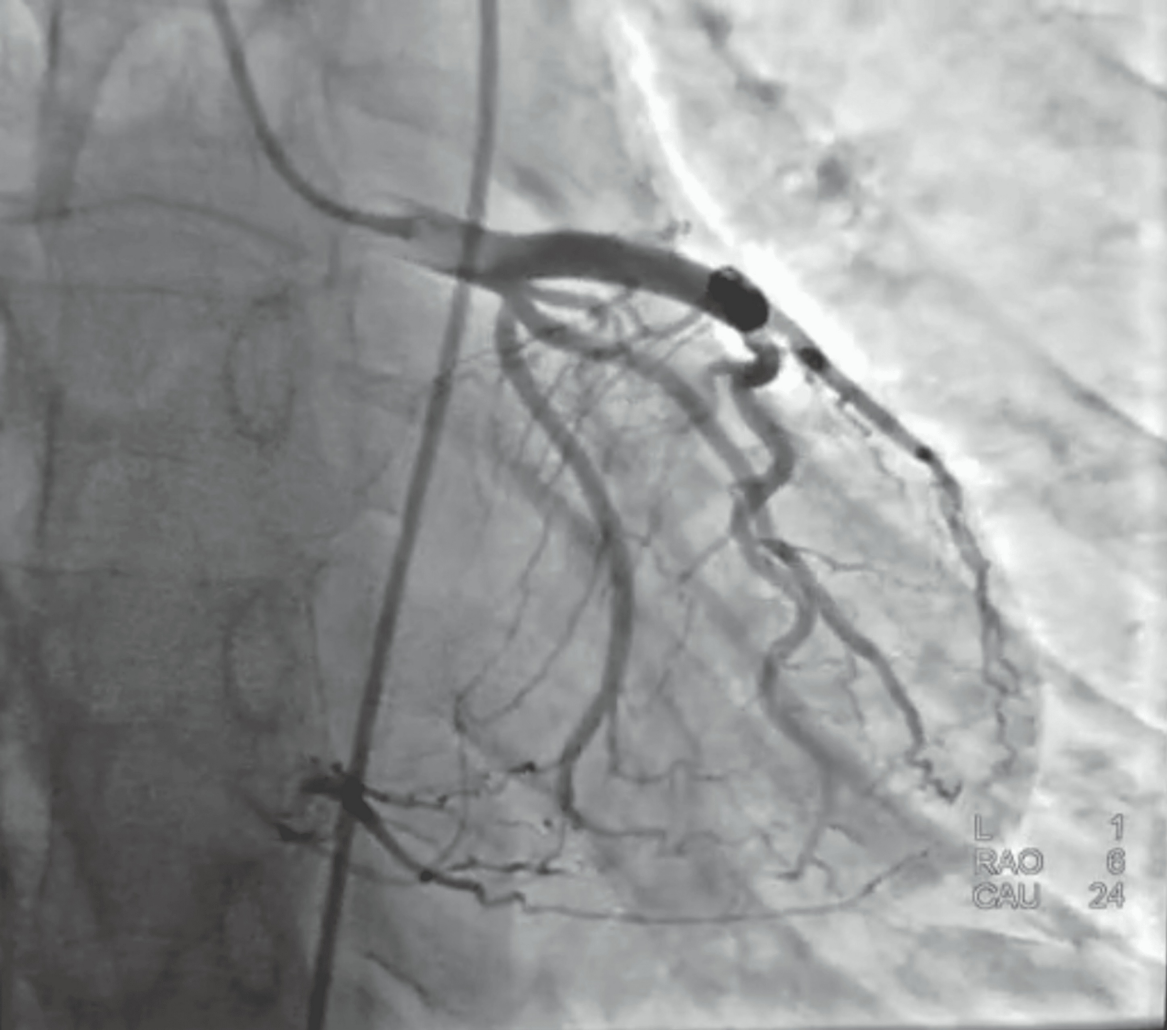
Coronary angiography: Mid-LAD plaque and chronic RCA occlusion correlating with ischemic MRI findings. Collateral circulation supplying the RCA territory from the LAD. Images were obtained from the authors’ institutional database. LAD, left anterior descending; RCA, right coronary artery; MRI, magnetic resonance imaging
